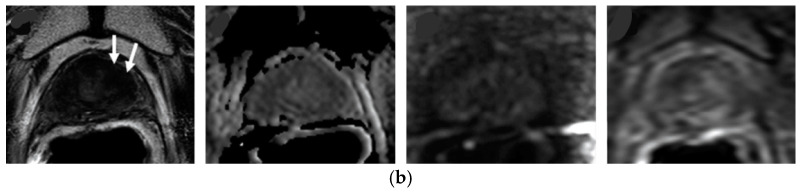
A 55-year-old man who was treated with external beam radiotherapy. Pre-treatment prostate-specific antigen—PSA level was 7.96 ng/mL, and 1 year after treatment, PSA level was decreased in 0.76 ng/mL. (a) Original tumor on transition zone (arrow) on Axial T2weighted Imaging (T2WI), apparent diffusion coefficient (ADC), b-2000 diffusion weighted (DW) and diffusion contrast enhancement (DCE) images (from right to left) (b) The 1 year posttreatment follow-up observed decreased prostate size, lower DWI signal and no focal enhancement on DCE imaging.
